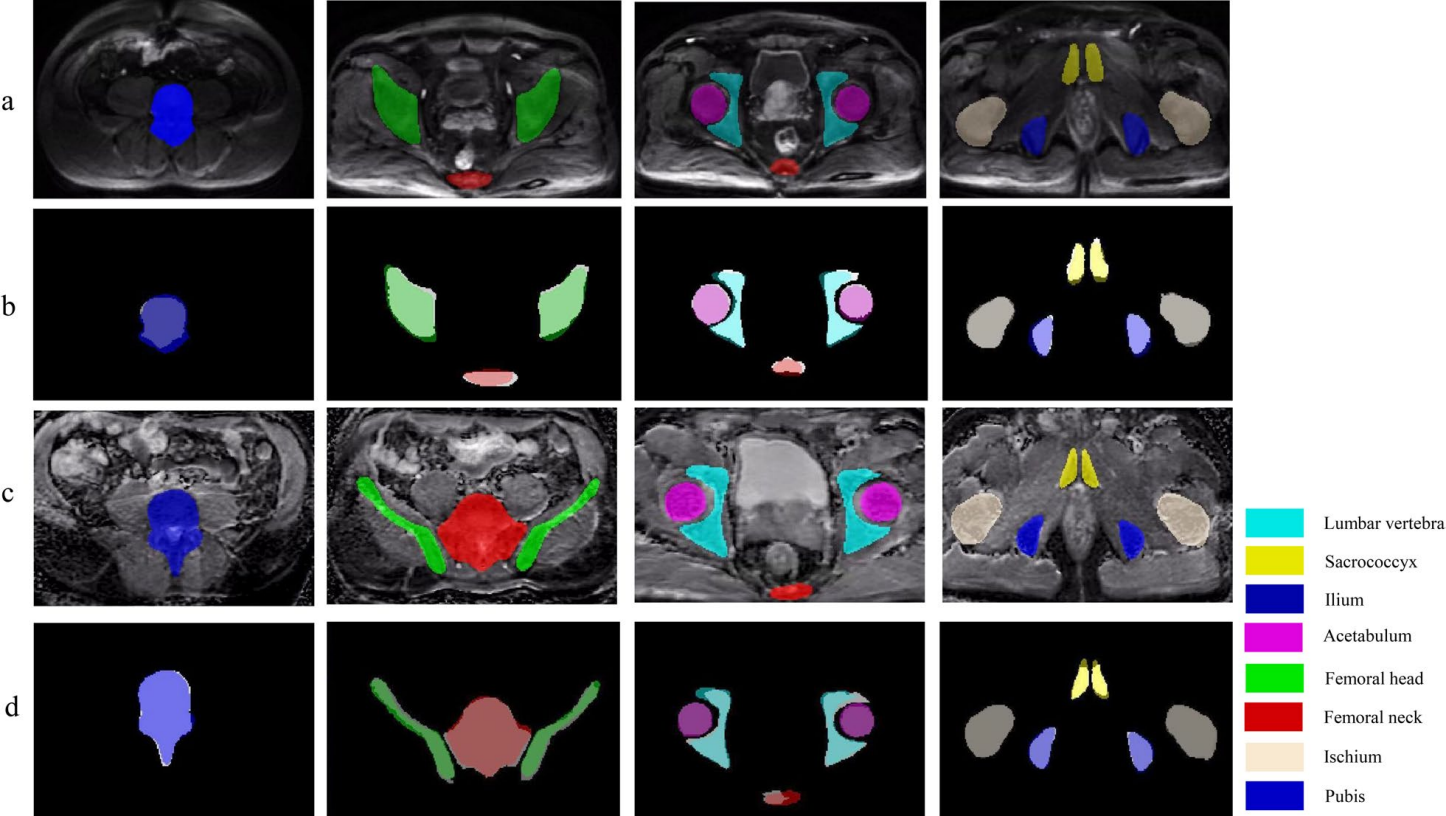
Examples of the comparison between CNN-predicted and manual segmentations. a Section examples of eight bones on DWI image; b The corresponding overlapping images between manual segmentation (white background) and CNN-predicted segmentation; c Section examples of eight bones on ADC image; d The corresponding overlapping images between manual segmentation (white background) and CNN-predicted segmentation
